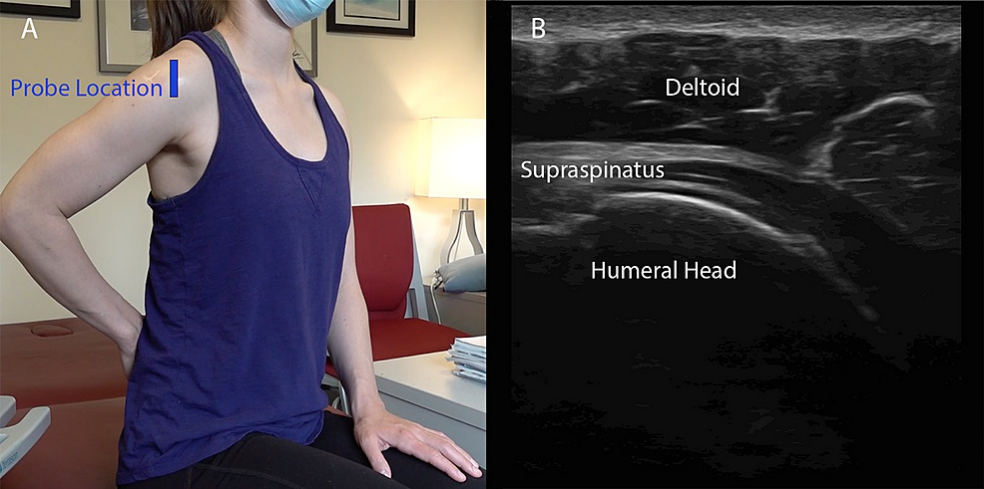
Supraspinatus (A) Demonstration of Crass positioning which will best expose the supraspinatus tendon. The dorsal aspect of the hand faces the patient’s lower back and the location of the ultrasound probe in order to obtain a long-axis view of the supraspinatus is shown. (B) The acquired long-axis view of the supraspinatus tendon. The probe may be rotated 90 degrees clockwise to obtain a transverse view of the tendon.
